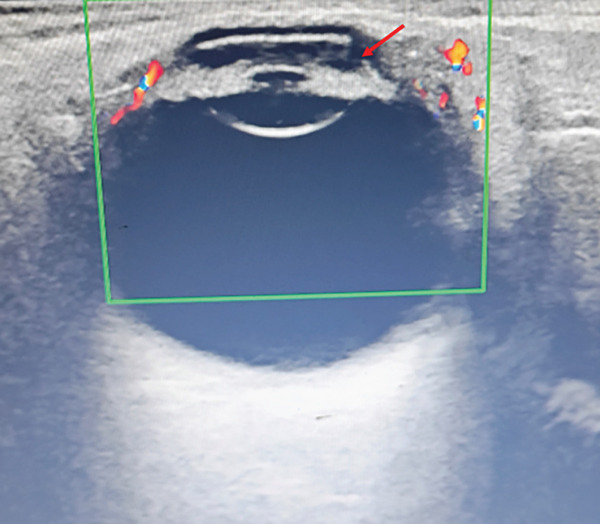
Ultrasound imaging of the left eye prior to emergency intervention. Note the presence of severe anterior chamber clouding (arrow in red) and the absence of significant vitreous abnormalities.
